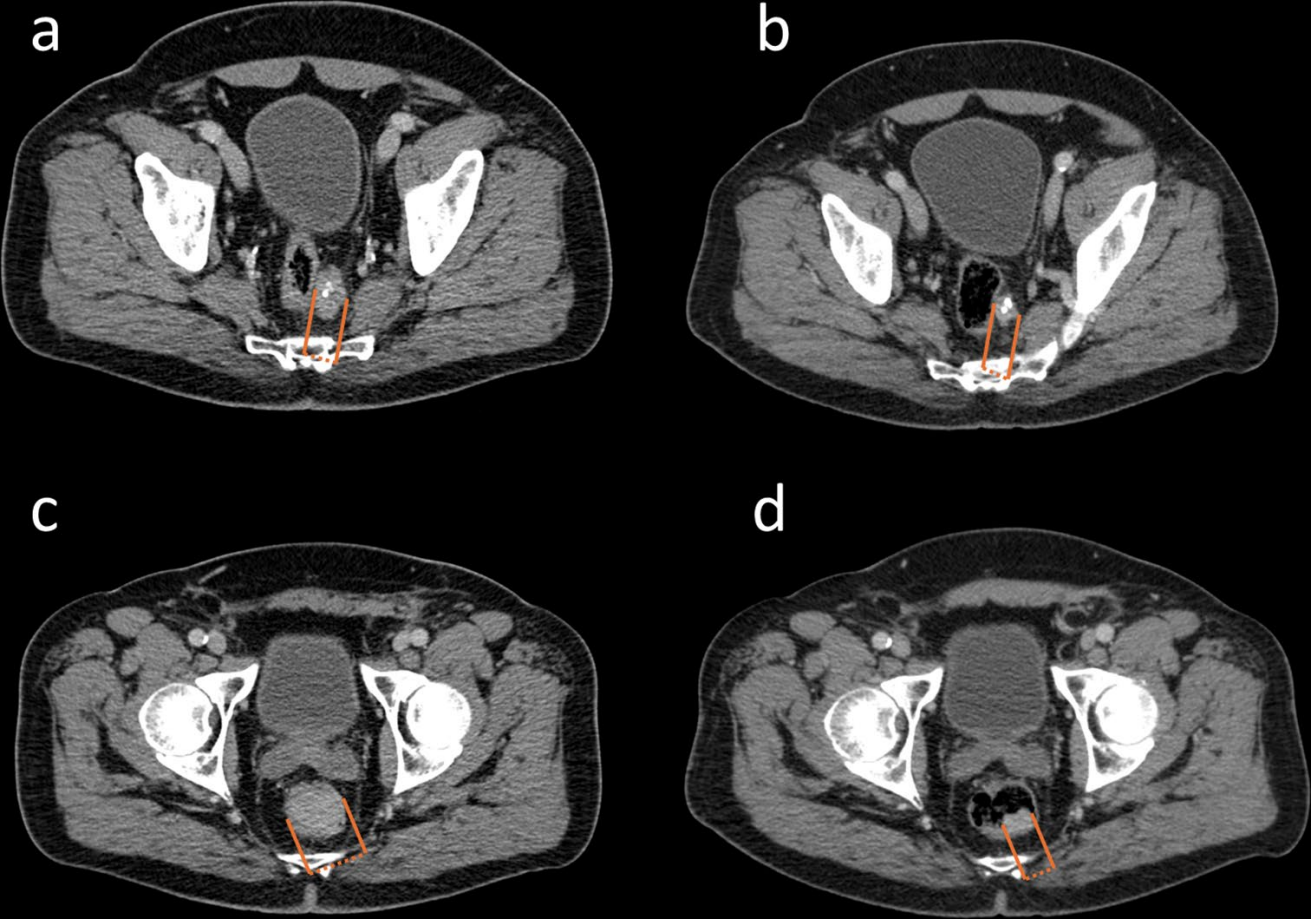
CT-images at baseline (a/c) and after 4 cycles of PRRT (b/d) of a patient with favorable risk factors at baseline (De Ritis ratio: 0.63, CgA: 14 µg/L, and ALP: 63 U/L), who had a partial response to PRRT and showed an OS of 64 months
